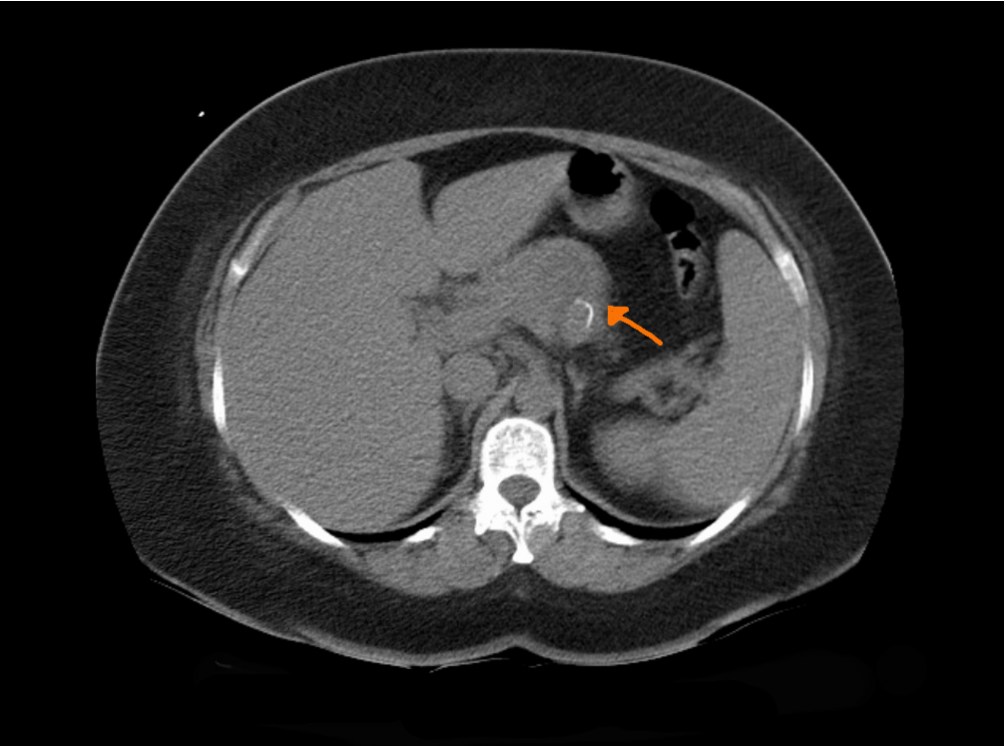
CT scan showing lesion in the pancreas.
